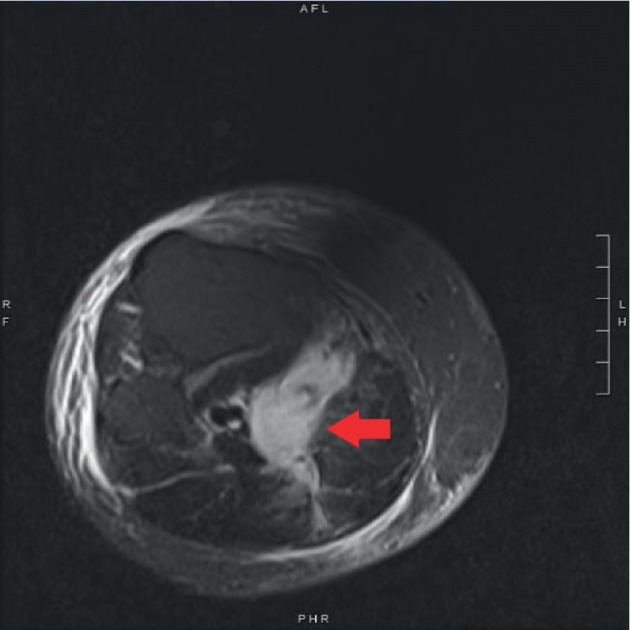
MRI imaging demonstrating complex fluid in the semimembranosus-gastrocnemius bursa (red arrow) with associated edema. Surrounding fluid extravasation is representative of ruptured Baker's cyst. Associated subcutaneous, muscular, and fascial edema is visualized.
